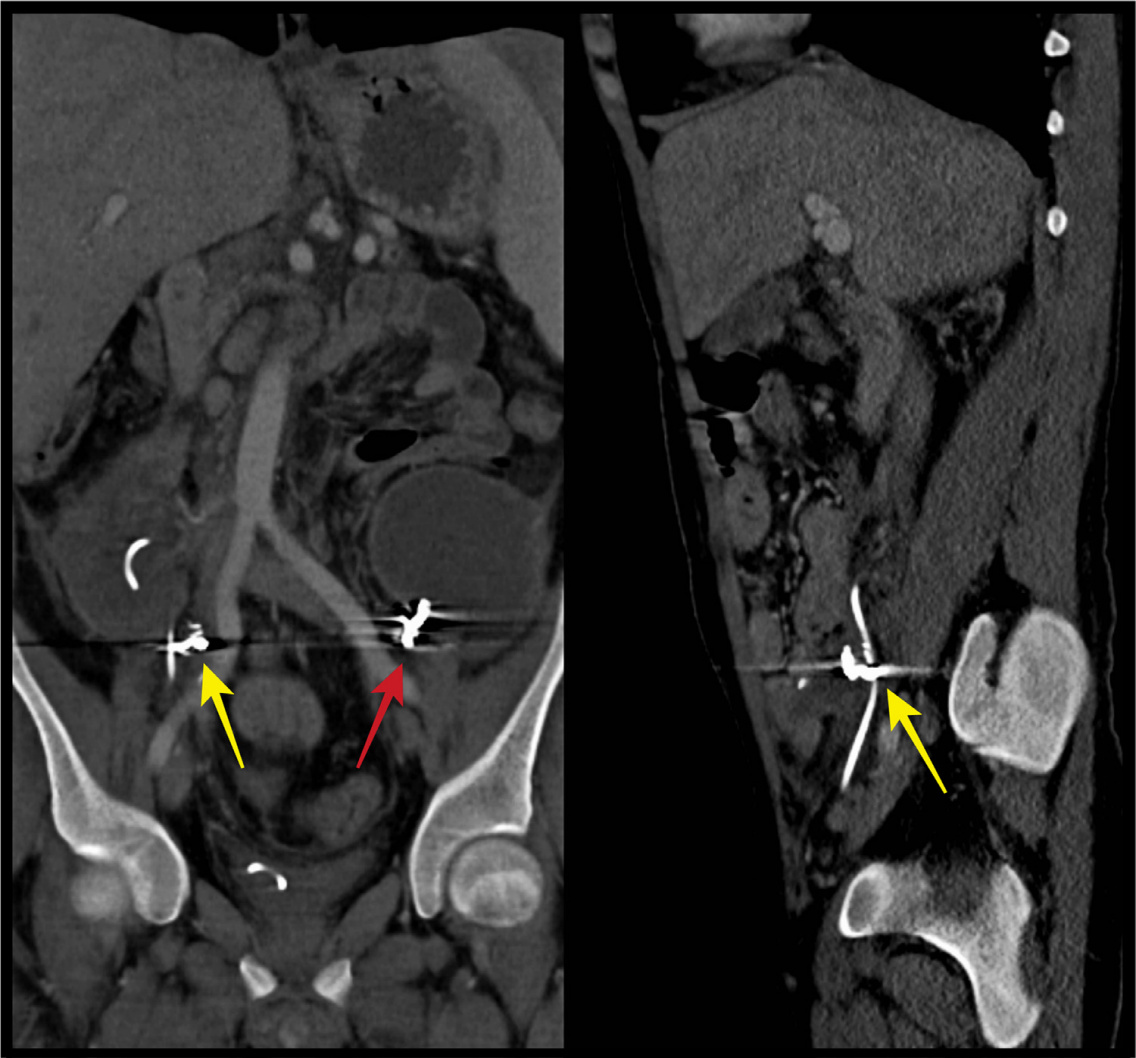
Coronal and Sagittal CT imaging of the patient's abdomen and pelvis following AUF embolization. Red arrow redemonstrates the embolization coils from the preceding operative embolization of the right renal transplant that were previously identified in Figure 1. Yellow arrows identify the newly deposited embolization coils within the coronal and sagittal images. The coils were implanted within the fistulous tract between the pancreatic transplanted artery and the right ureter.
